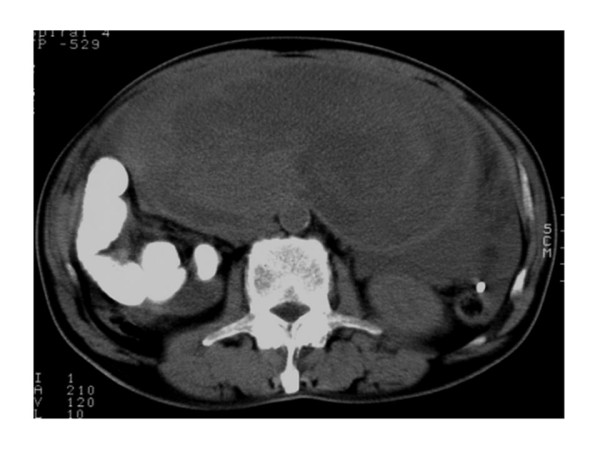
Emergency CT revealed reduction of the tumor and retention of ascites. Arrows: past contrast medium used gastroenteric examinations.
